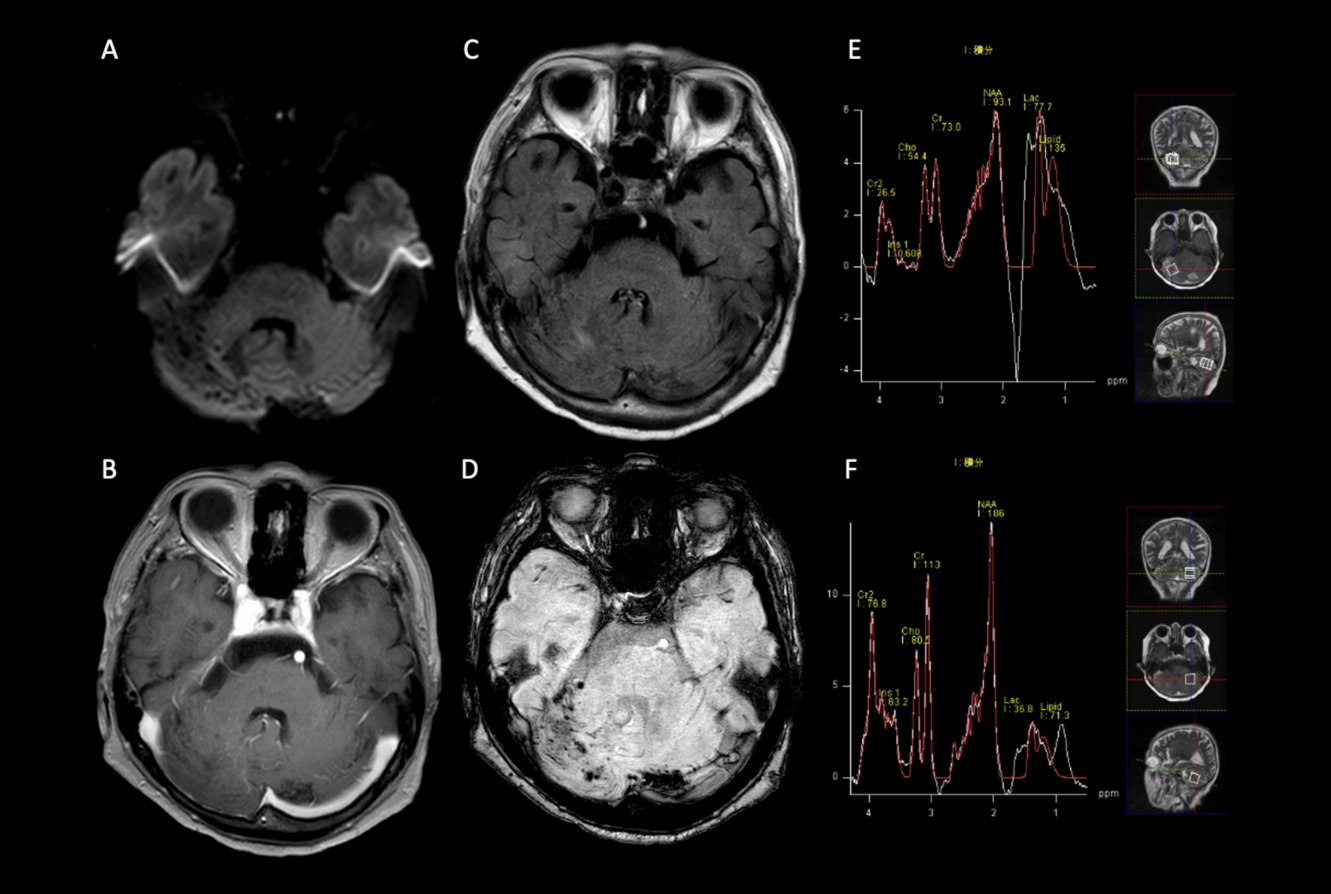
MRI 10 months after vascular treatment. Shrinkage of contrast-enhanced lesions, cerebellar hemisphere edema, and venous dilation on the right side are observed (A-D). However, there is no improvement in NAA and Lac levels which remain relatively high on the affected side (E, F). MRI: magnetic resonance imaging; NAA: N-acetylaspartate; Lac: lactate
